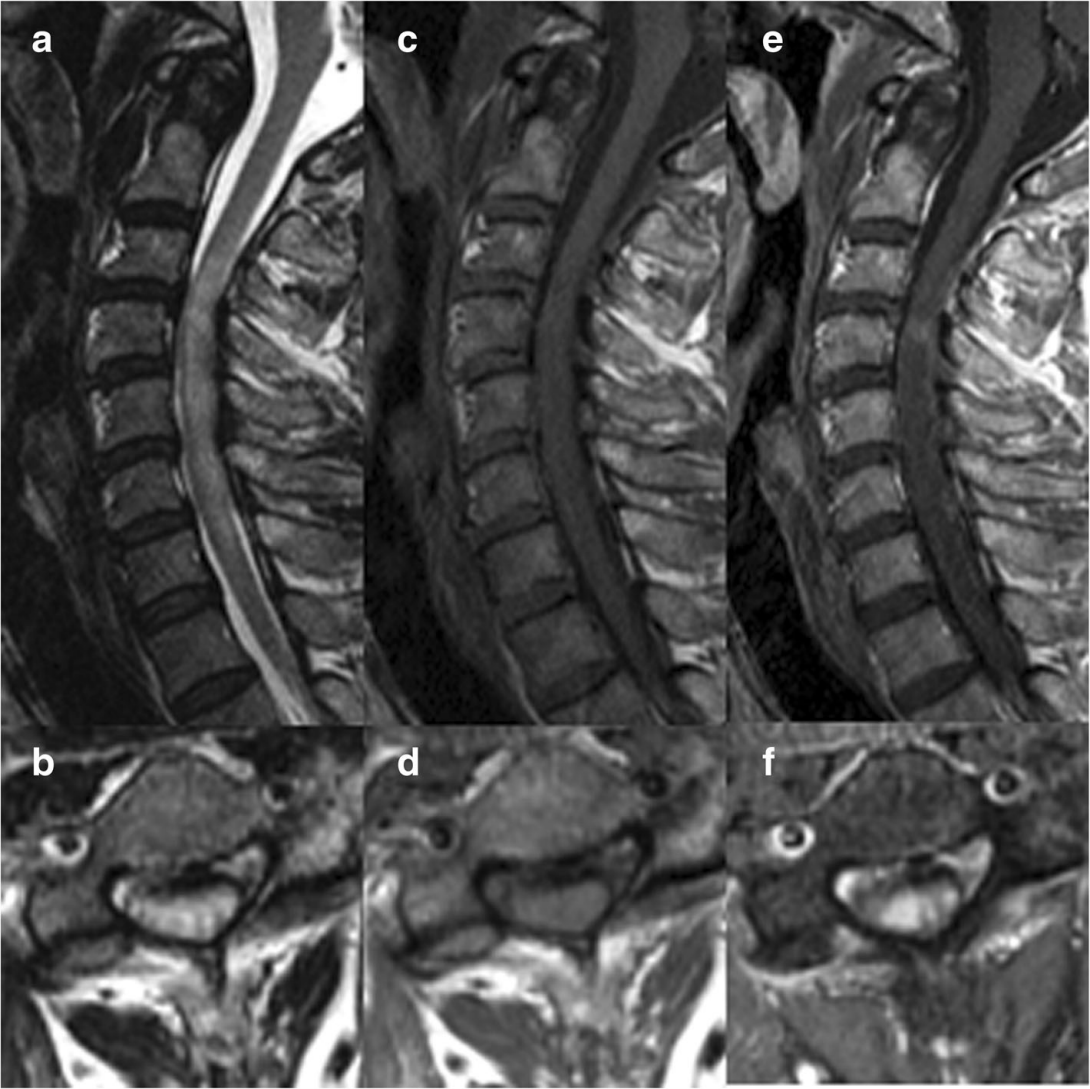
MRI images of a 53-year-old man with spinal cord edema due to cervical spondylosis revealing T2 high (a, b), T1 iso (c, d) signal intensity, and pancake-like gadolinium enhancement (e, f)
